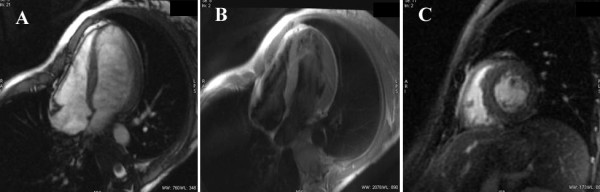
Cardiac-MRI images: (A) Cardiac MRI gradient echo sequence showing normal dimensions and function of the left ventricle. (B) T2-weighted short τ inversion recovery sequence showing the absence of edema. (C) Late gadolinium hyperenhancement sequence without pathological contrast captation.
